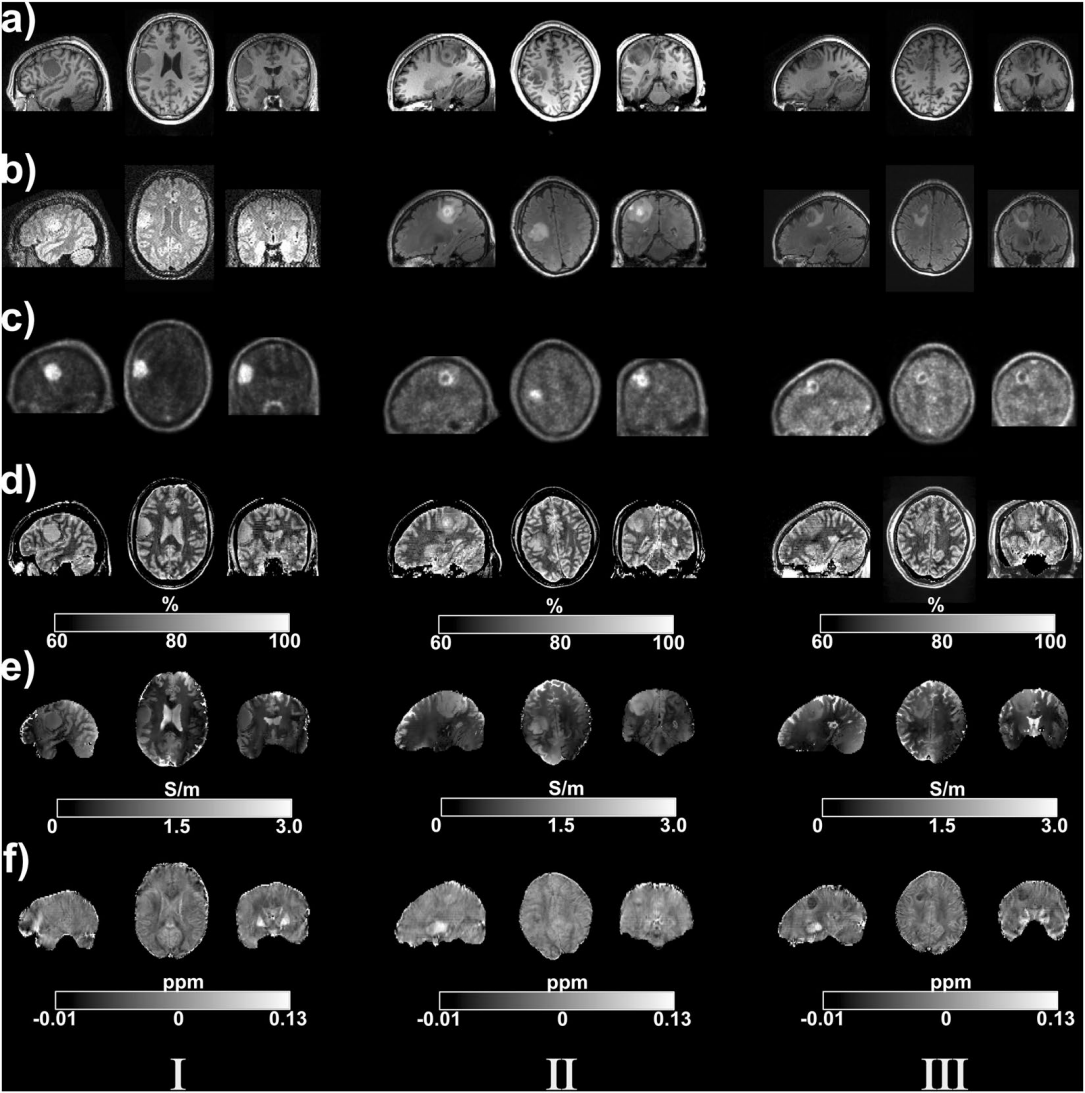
MR and PET images from three tumour patients. Sagittal, transverse and coronal slices through the tumours. (a) T_1_-weighted MPRAGE (b) proton FLAIR (c) FET-PET (d) quantitative water content (e) electrical conductivity and (f) quantitative susceptibility images. The colour bar indicates the water content in percentage, susceptibility in ppm and conductivity in S/m in the images.
